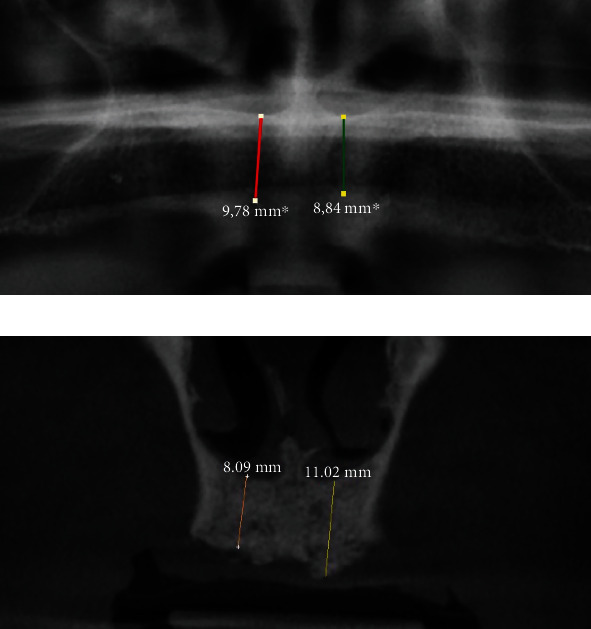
Measurement of the shortest vertical distance between the bottom of the nasal floor and the alveolar crest on a panoramic image (a) and a cone beam computed tomography image (b).
